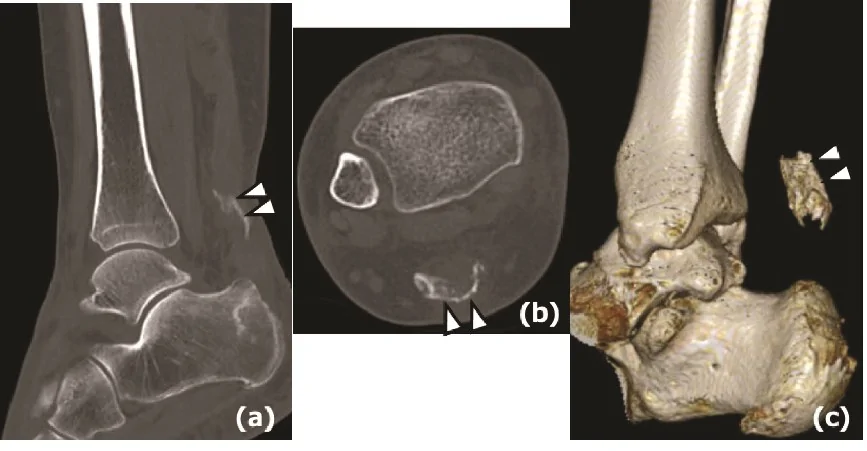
Preoperative CT, showing detailed fracture configuration and fragment size. (a) Sagittal image, (b) axial image, (c) 3D reconstruction.
